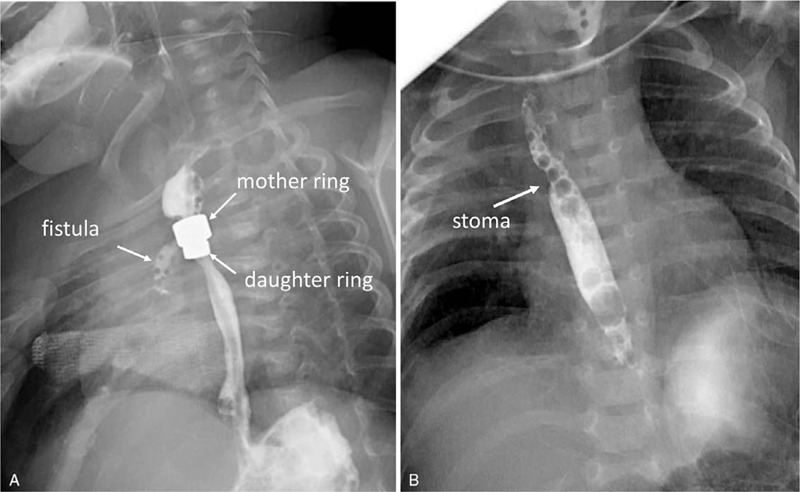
Serial postoperative radiographs of the X-ray images: (A) The esophagography confirmed the anastomotic fistula on day 7 after the surgery; (B) The esophagogram reveals that the magnet arrays were removed on day 36, and that the esophageal achieved restoration without leakage.
